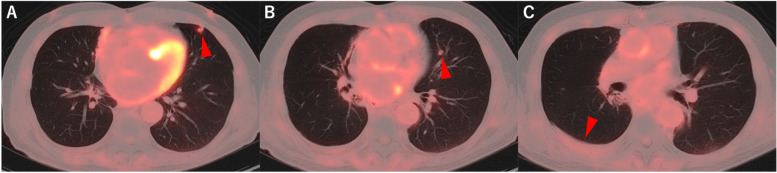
PET-CT at recurrence revealed an SUVmax of 3.4 for two small nodules in the left lung lingular segment (A, B). A small amount of right pleural effusion without abnormal uptake was also observed (C)
